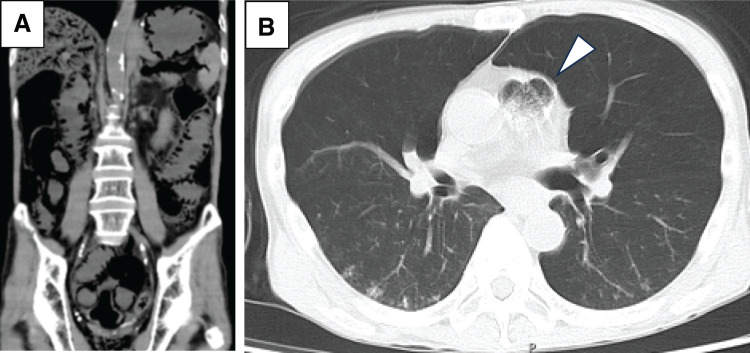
Images of CT at the second occurrence of PI and PVG. CT revealed more severe PI and PVG involving the entire length of the small intestine (A), with gas extending into the right atrium (arrow head) (B). PI, pneumatosis intestinalis; PVG, portal venous gas
